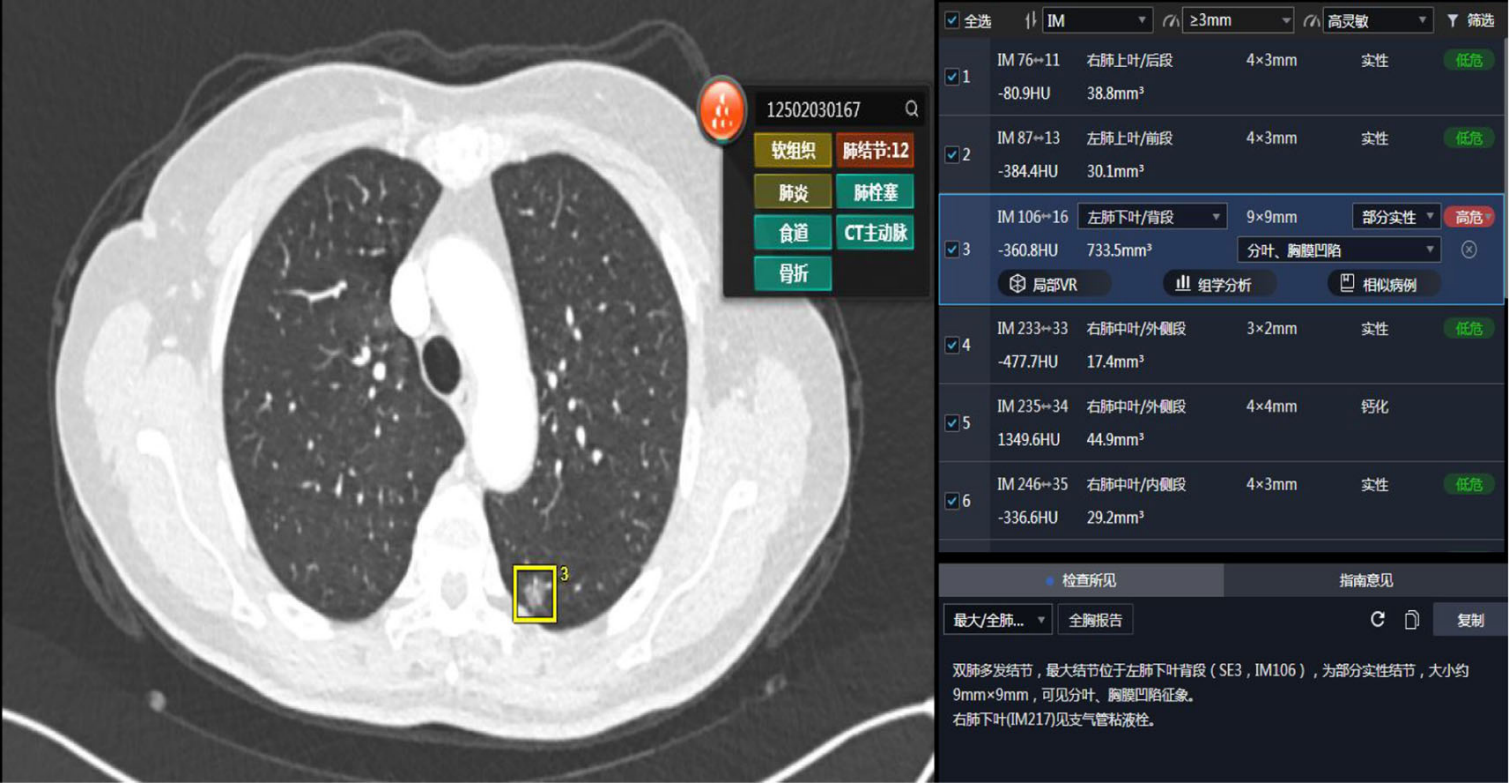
The uAI platform detects PN on thoracic computed tomography (CT) images and conducts artificial intelligence-driven risk stratification of the identified lesions.
